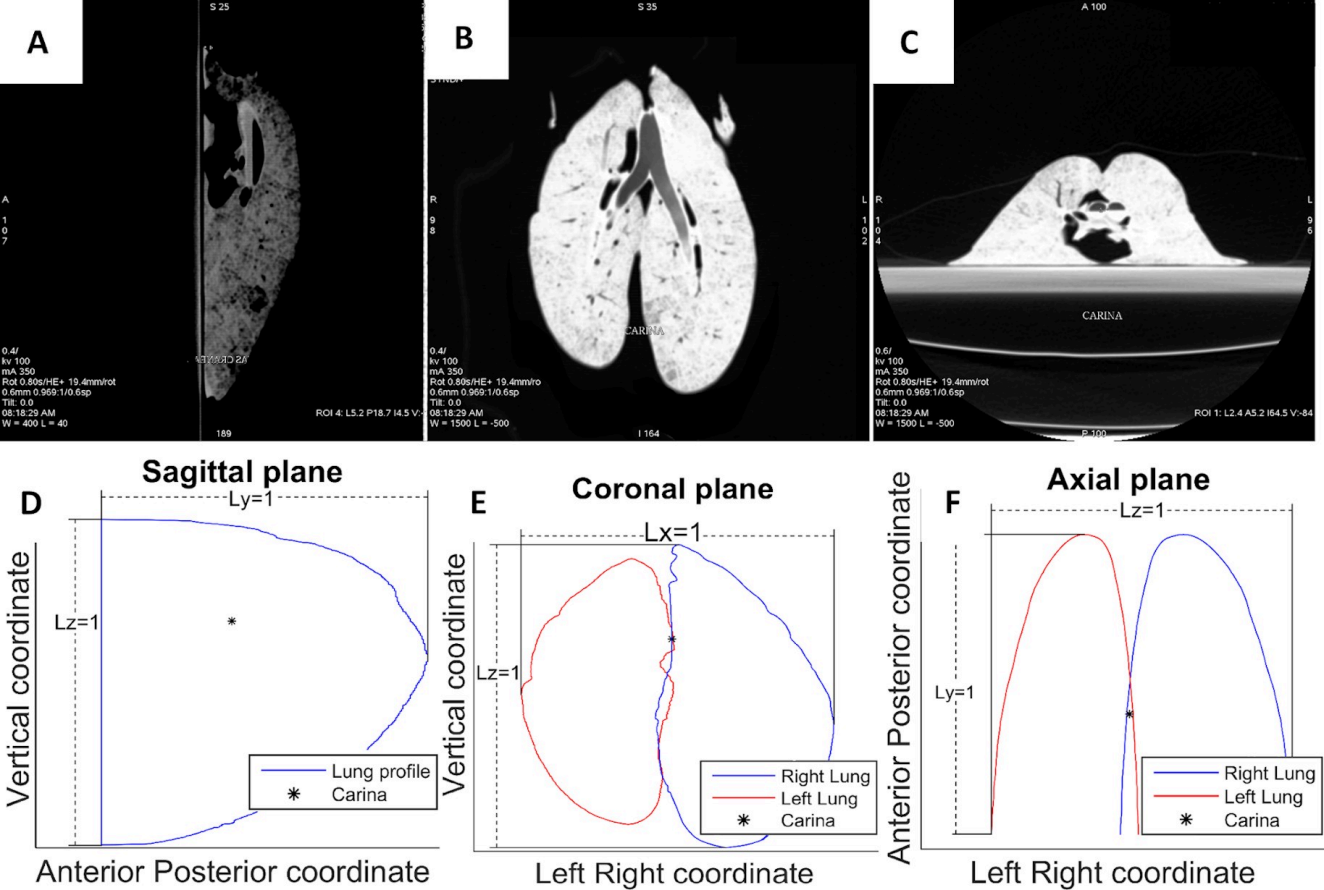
Normalized contour lines obtained from CT-scan images. Contour lines were obtained from CT-scan images and used for a 3D computational reconstruction of the pulmonary surface. Trachea division point (carina) is marked for purposes of reconstruction. (A) Sagittal plane CT image. (B) Coronal plane CT image. (C) Axial plane CT image. (D) Sagittal plane outline reconstruction. (E) Coronal plane outline reconstruction. (F) Axial plane outline reconstruction.
